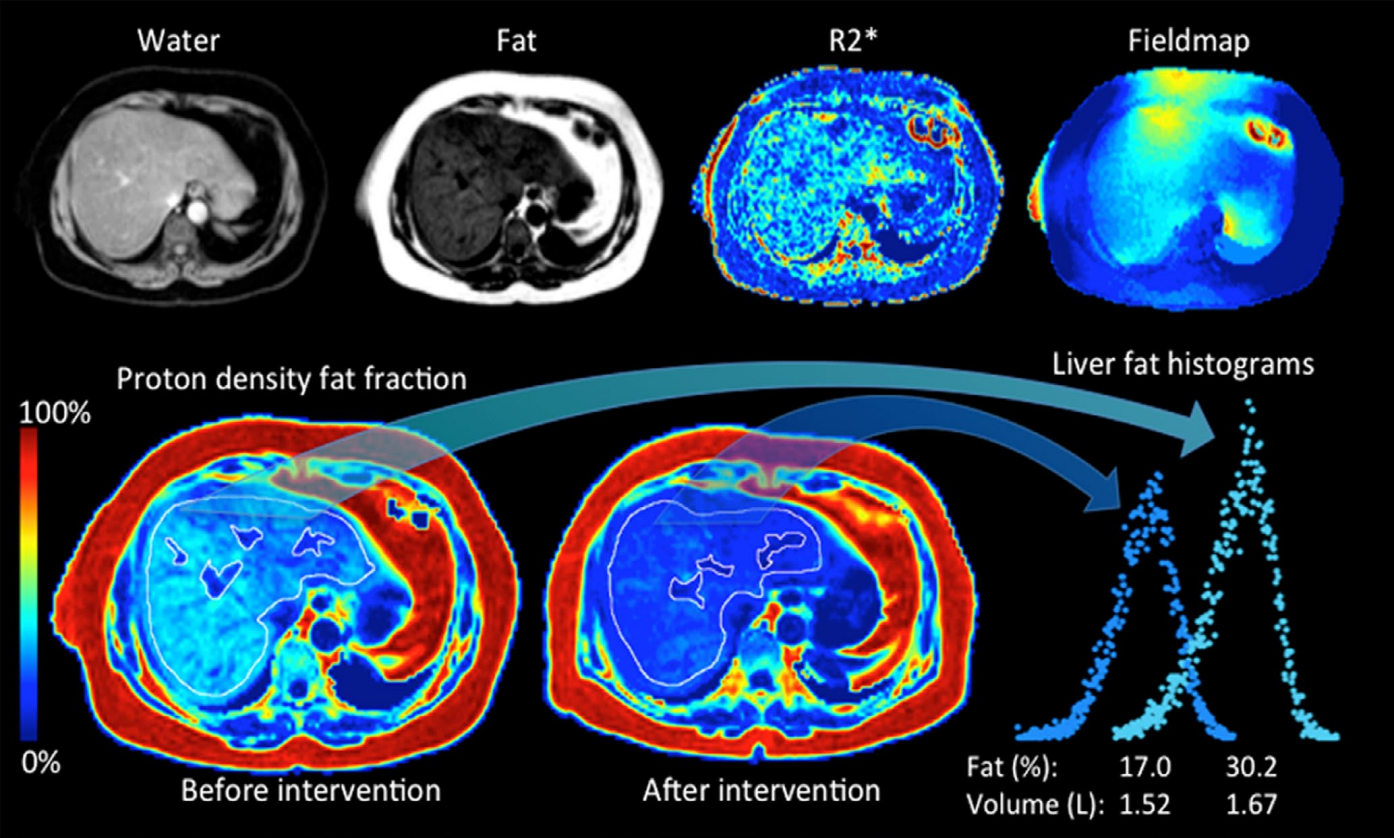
An example of a MRI‐PDFF map from the same patient before and after intervention. In this case, the entire liver is measured excluding major bile ducts and veins to improve precision. Here, also the liver volume was measured and reductions both in PDFF and liver volume are seen. The whole liver analysis also enables the histogram display and analysis of pixels throughout the liver as shown in the figure
